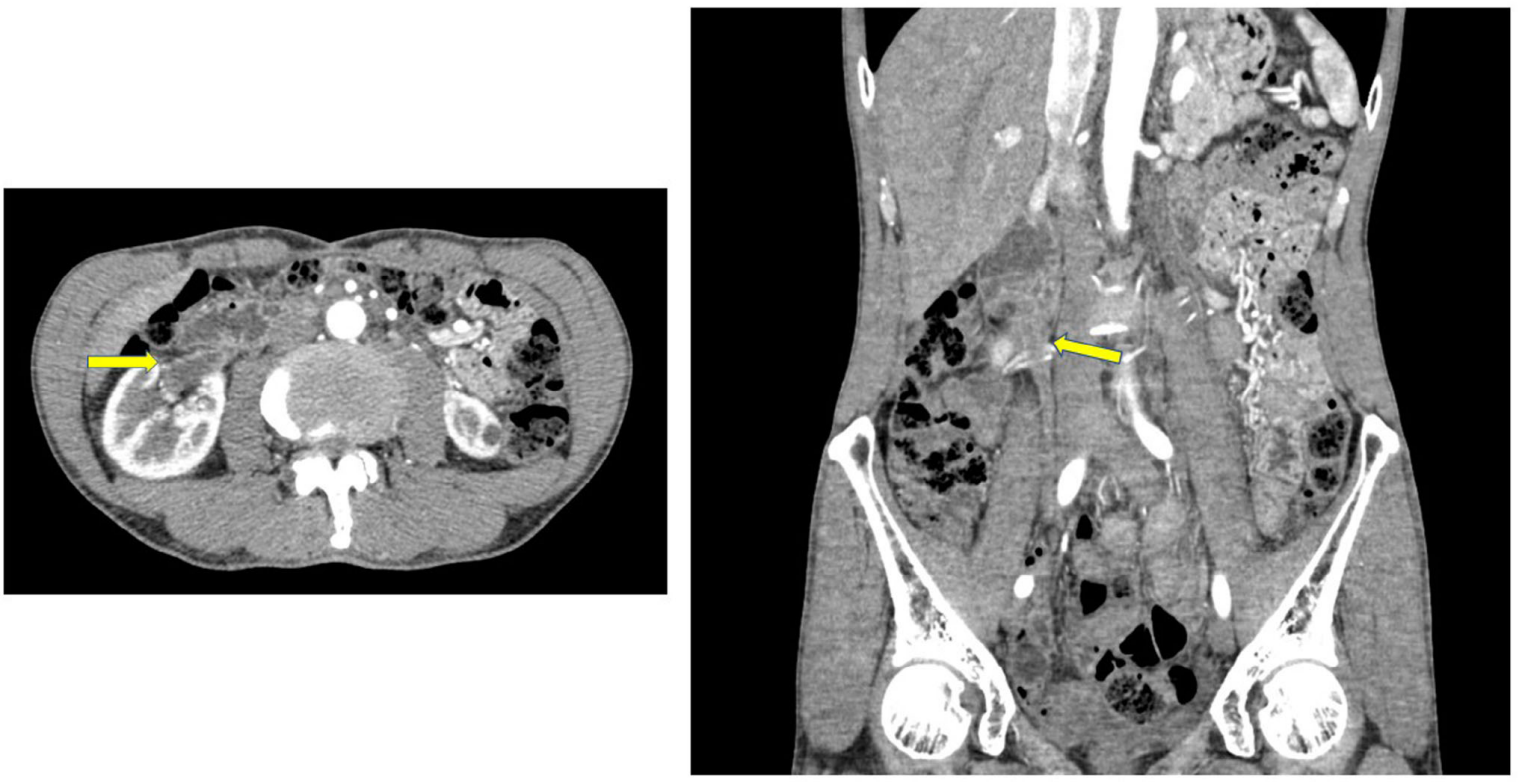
Contrast‐enhanced computed tomography scan showing dilatation, wall thickening, and irregularity of the right renal pelvis and upper ureter (arrow).
